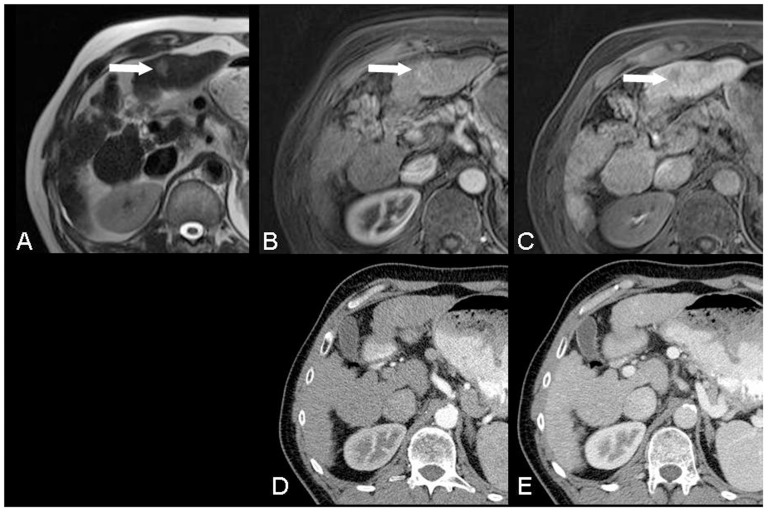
A 59-year-old male patient with liver cirrhosis and HCC (arrow) in S3 was only observed using MRI: *A*) markedly hyperintense HCC in T2w-HASTE axial, *B*) typical arterial enhancement in T1w-VIBE, and *C*) hypointense lesion in the hepatobiliary phase. No lesion was detected using CT: *D*) early arterial phase and *E*) portal venous phase.
